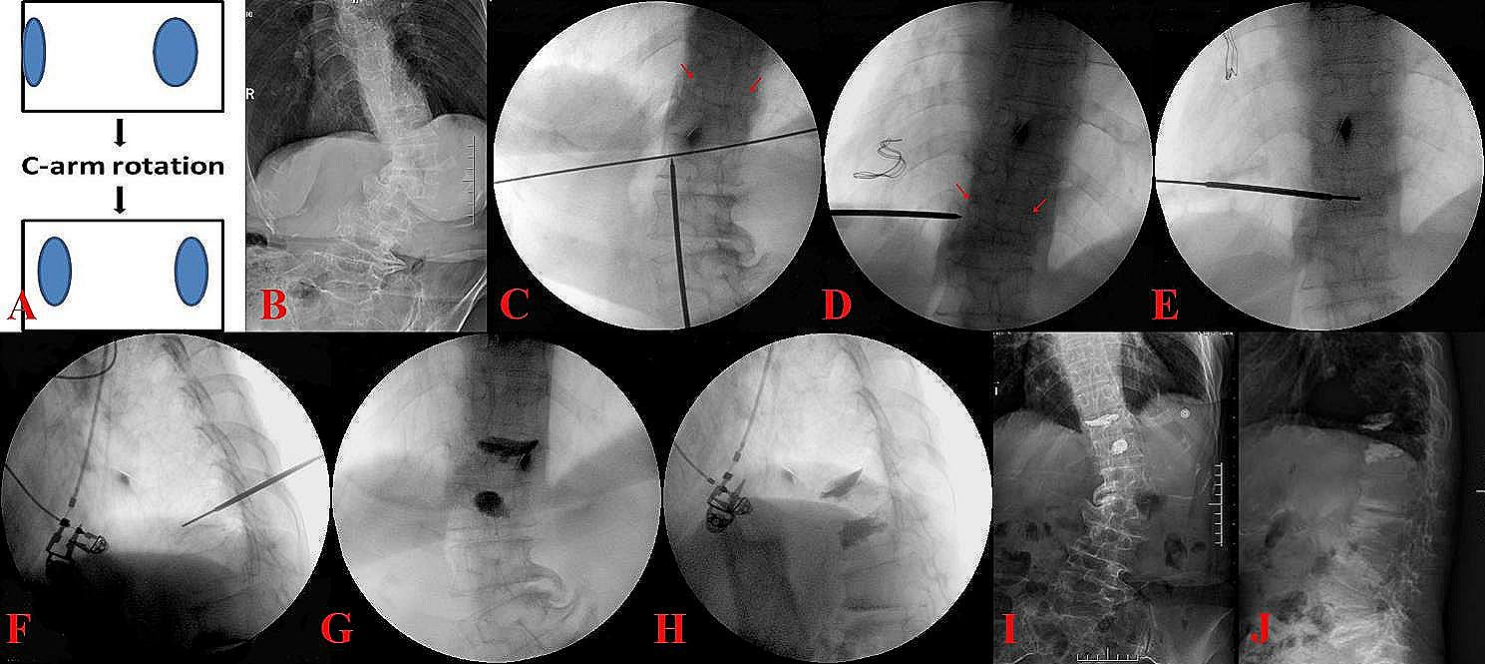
A, C-arm is gradually rotated until a true PA image of the rotated vertebral body is obtained and both pedicles are mostly symmetrically visualized en face; B, the X-ray of PA view at pre-operation; C, the intraoperative PA image revealed asymmetrical pedicle shadows (red arrows); D, C-arm position is modified and gradually rotated to obtain a true PA image of the rotated vertebral body and both pedicles are nearly symmetrical (red arrows); E and F, the guide wire was at or cross the midline in PA view (E) and reached the anterior one-fourth of the vertebral body at the lateral view (F); G and H, the final intraoperative PA (G) and lateral (H) images; I and J, the PA (I) and lateral (J) X-ray after surgery
